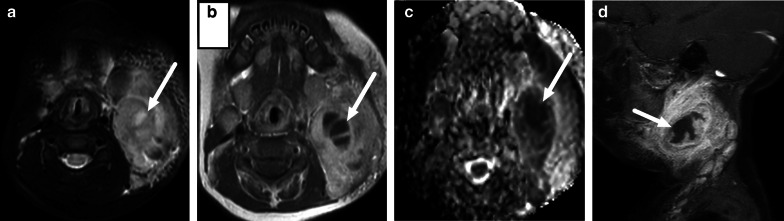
Lateral superficial suppurative lymphadenitis in an infant. Lymphadenopathy and surrounding edema are shown on an axial fat-suppressed T2-weighted image (a), and a non-enhancing center on post-contrast axial (b) and fat-suppressed sagittal (d) T1-weighted images with low ADC (c) (arrows). Imaging was consistent with suppurative lymphadenitis. Purulence was found during surgery and *Staphylococcus aureus* in pus cultures. Image modified from ref. [8]
